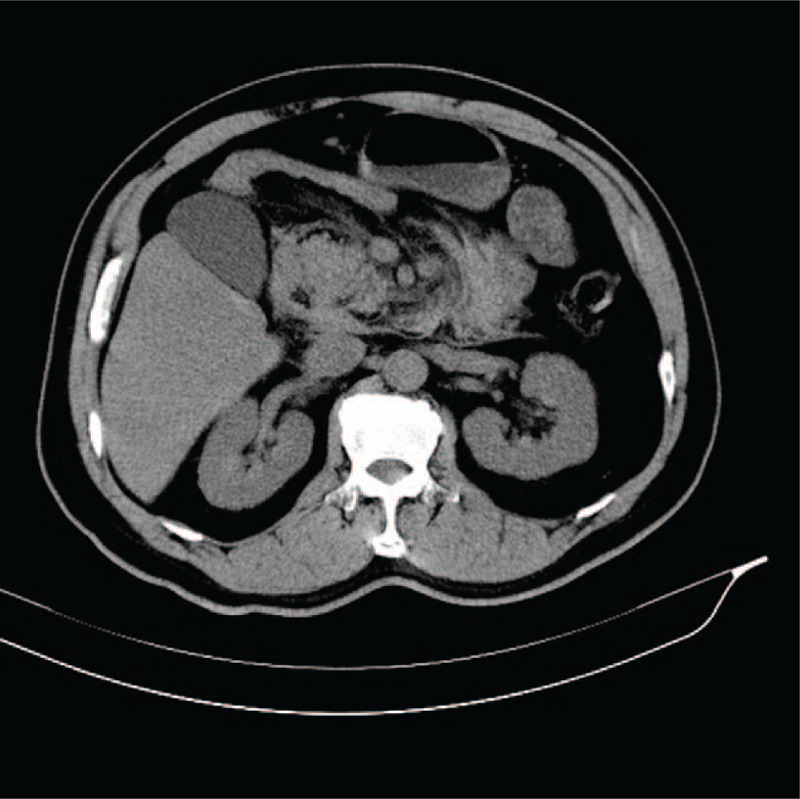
Computed tomography scan showing enlargement of the pancreatic head, as well as infiltraion of peripancreatic fat.
